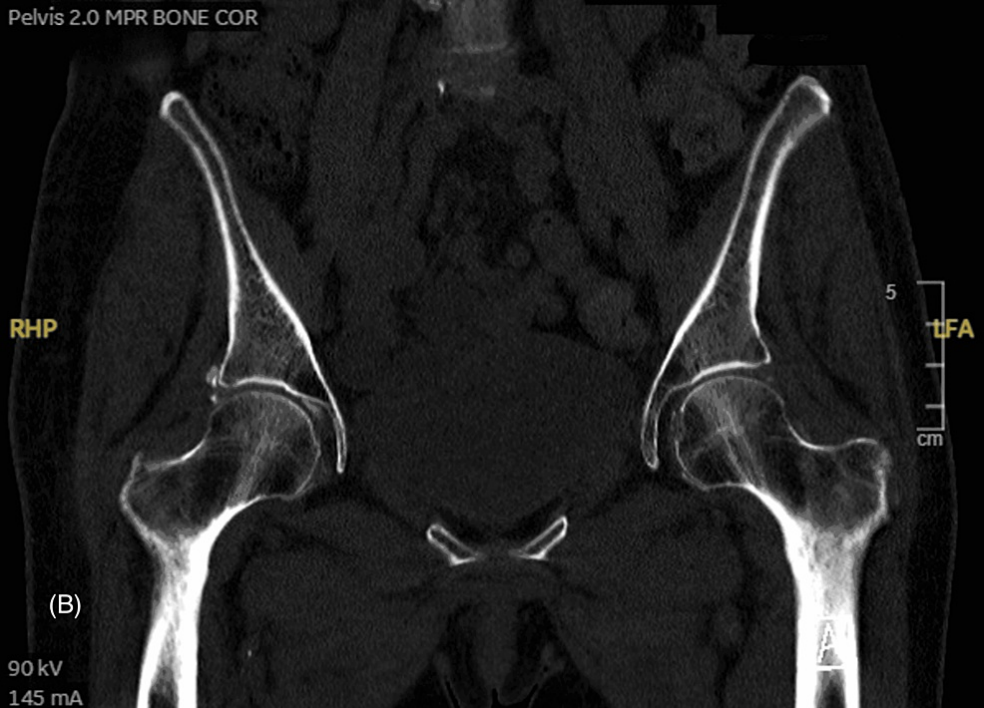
CT scan of the pelvis without contrast coronal image CT: computed tomography
